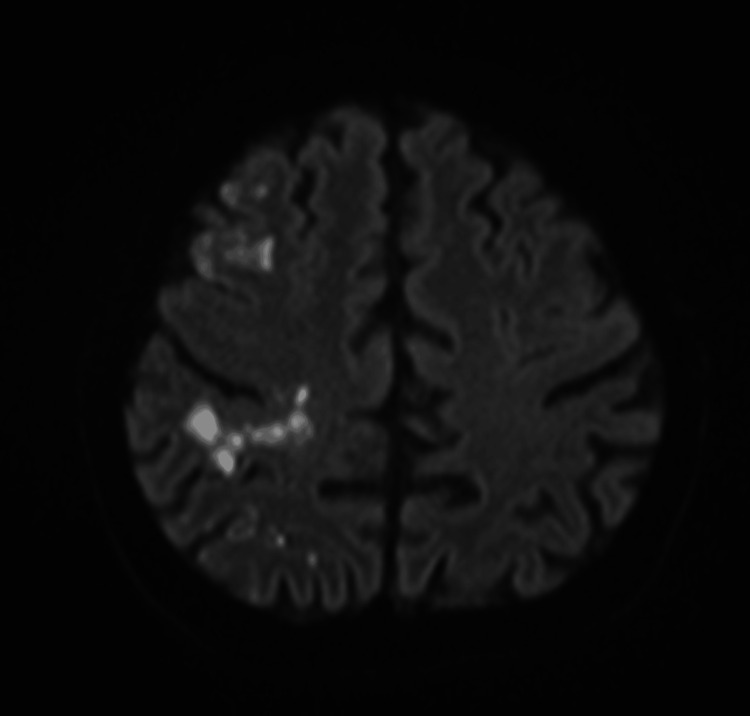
Magnetic resonance imaging diffusion-weighted imaging sequence demonstrating areas of restricted diffusion consistent with multiple acute ischemic infarctions in the right middle cerebral artery territory.
